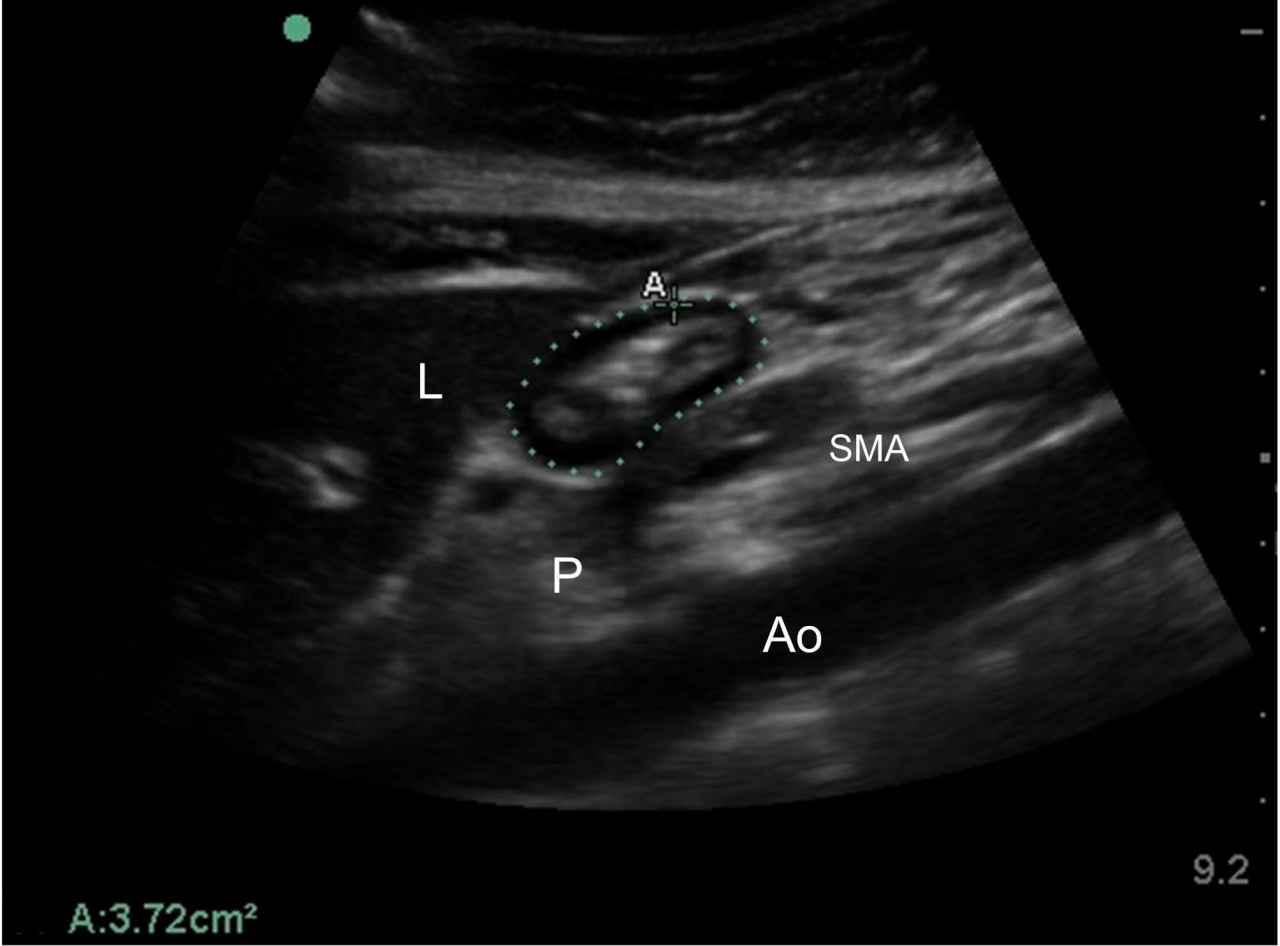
Example of gastral antrum images on ultrasonography. A, antrum of stomach; L, left lobe of liver; Ao, descending aorta; SMA, superior mesenteric artery; P, pancreas
